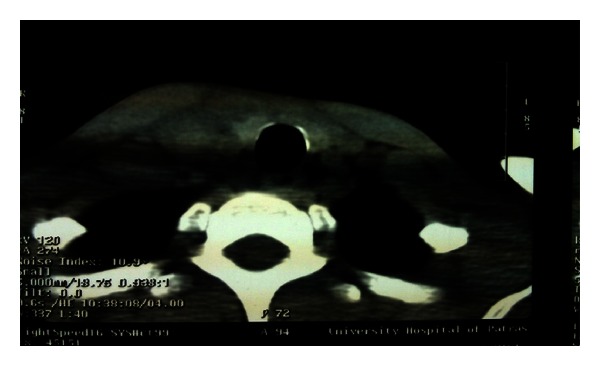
CT showing thrombosis in the right internal jugular vein and soft tissue.
